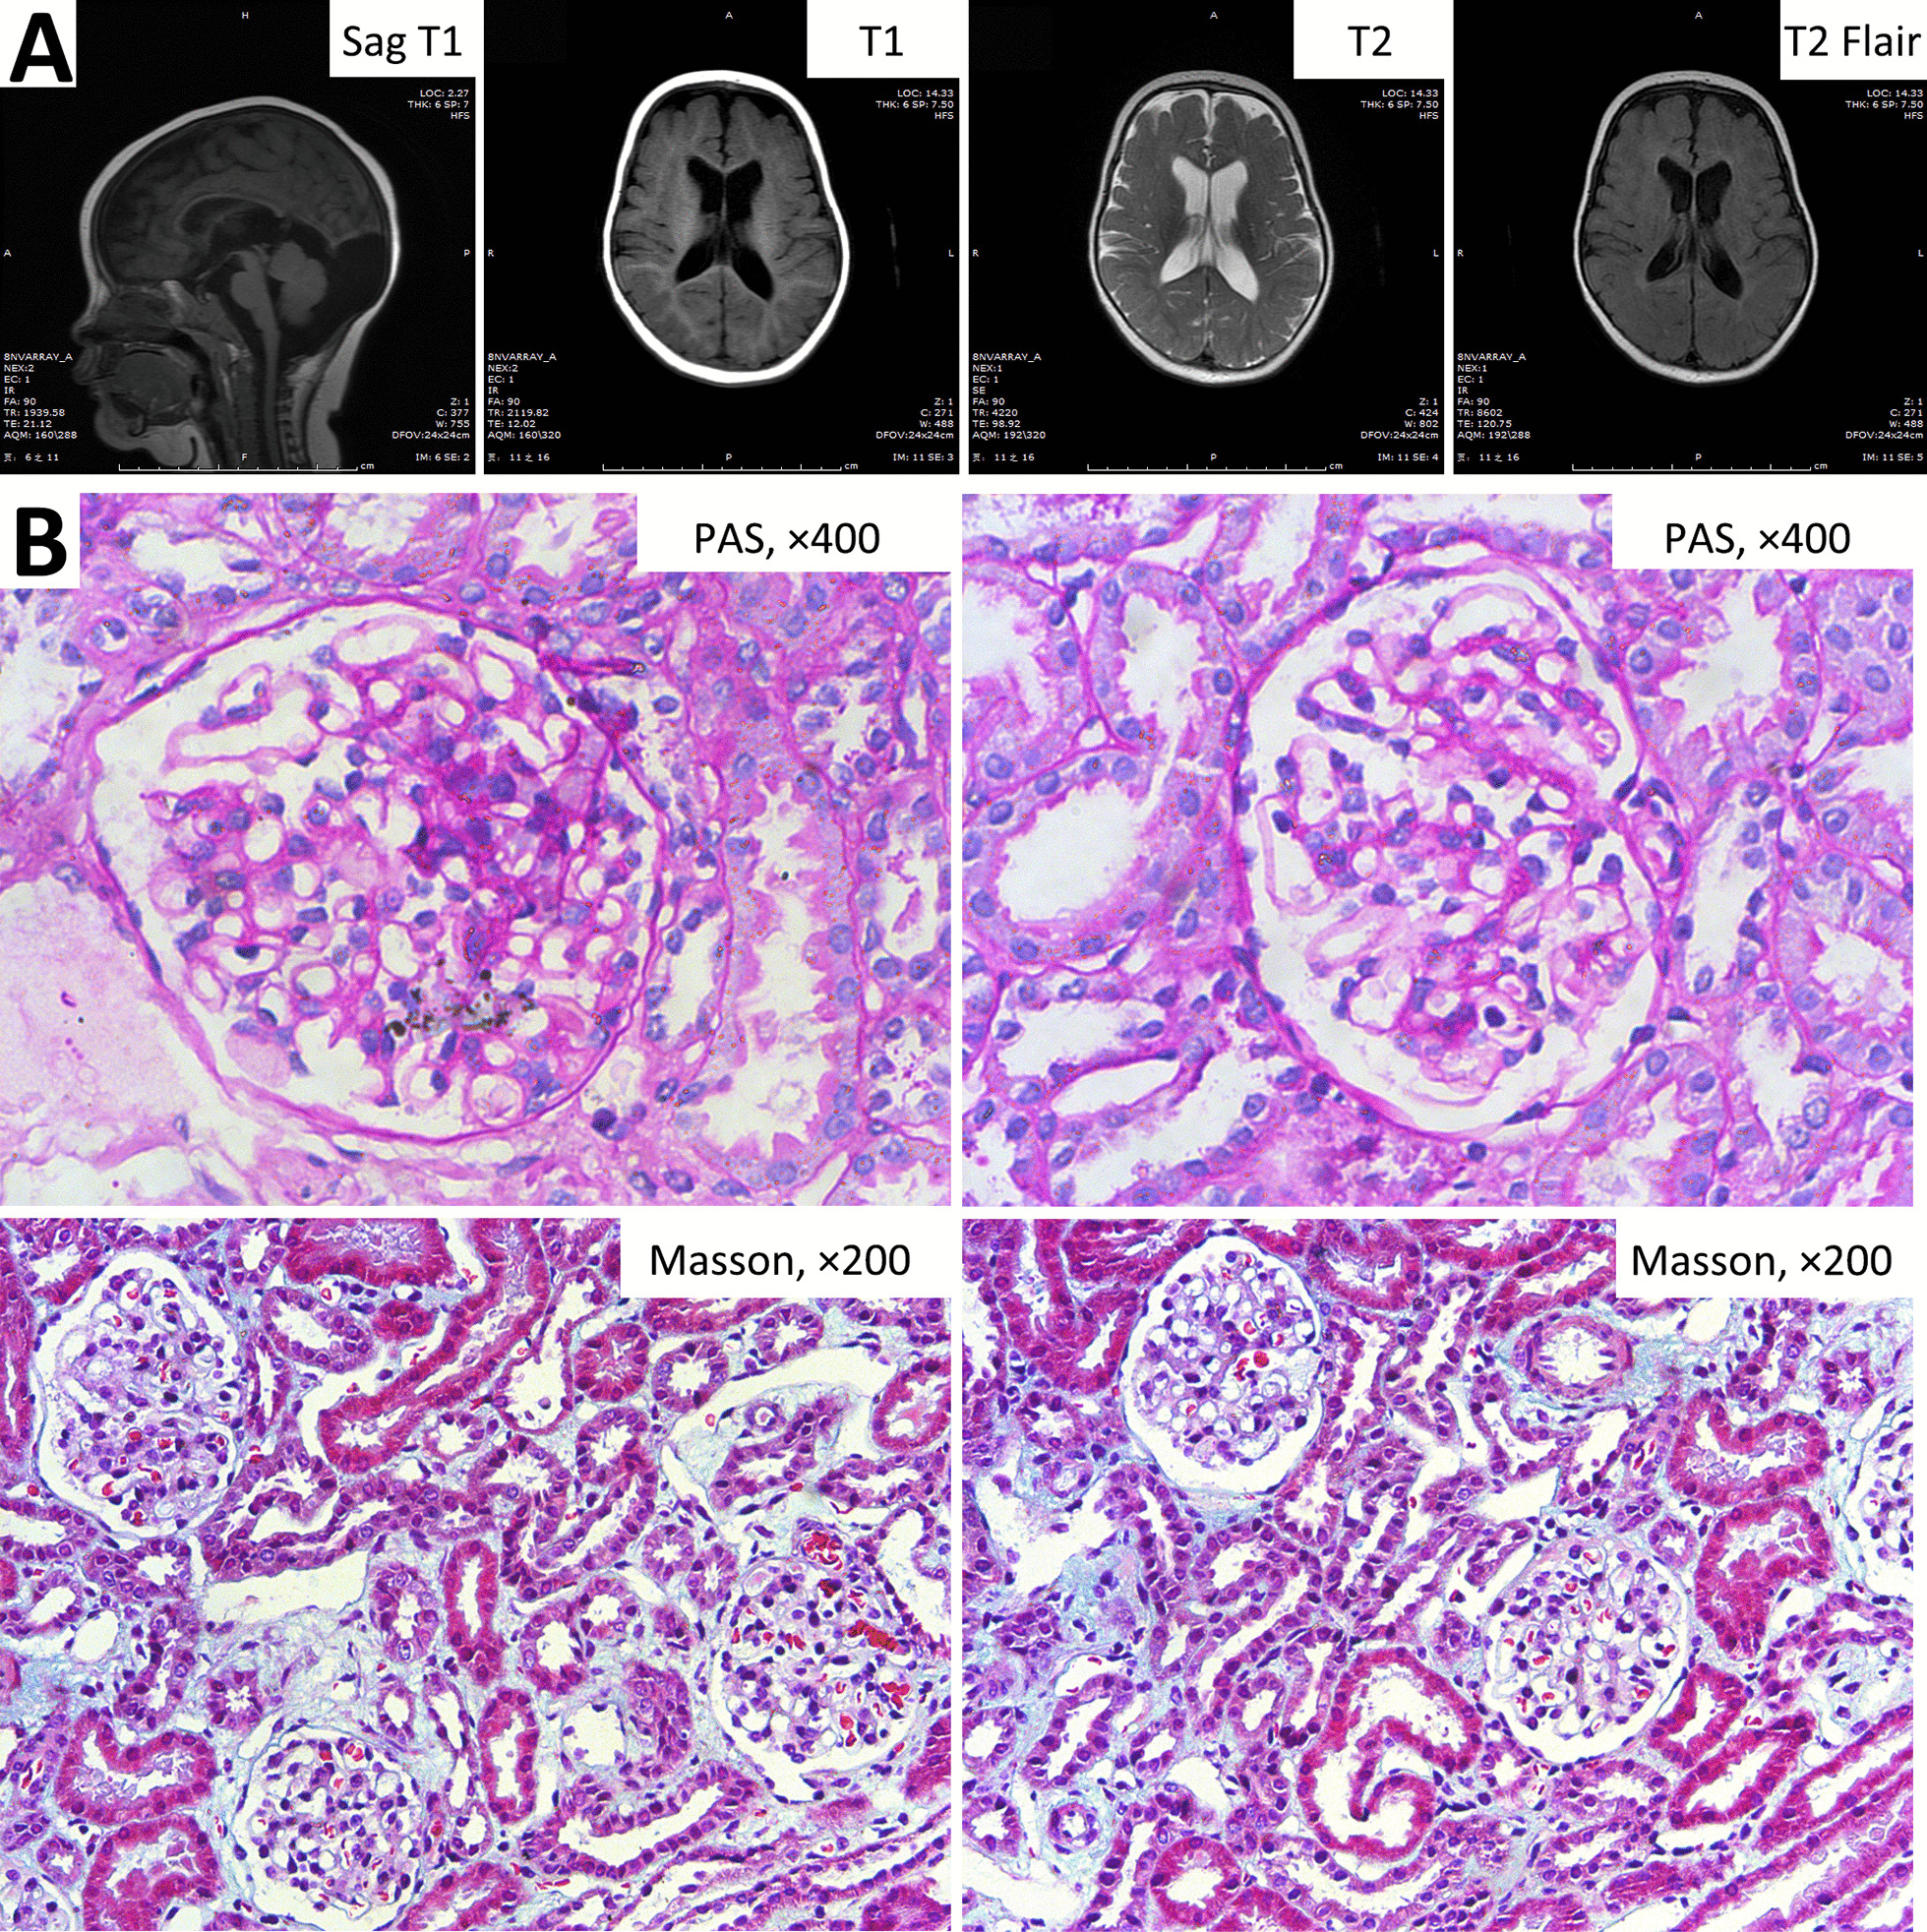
Brain MRI and renal pathological changes of patient 2 at the age of 7 months. a Note the presence of ventriculomegaly, white matter shrinkage, leukoaraiosis, cerebellar hypoplasia, and a large cistern magna cyst. b Note the presence of interstitial focal fibrosis with infiltration of focal mononuclear cells and mild mesangial proliferation
